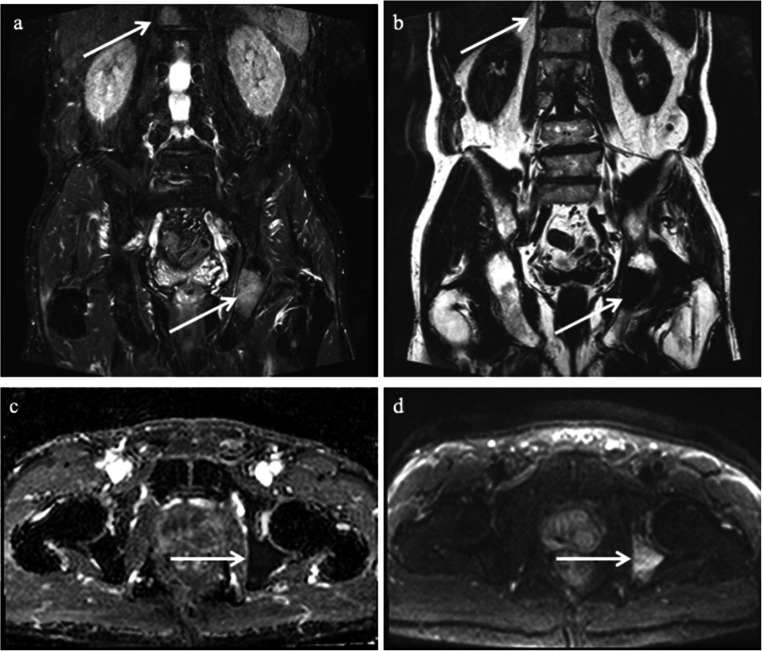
An 81-year-old patient with cT2/radT3a, PSA 9.5 ng/ml, and ISUP 3. The arrows indicate bone metastases in the left ischial body and the 12^th^ thoracic vertebral body. The prostate biopsies demonstrated a 90% Gleason grade 4 pattern in all ten biopsy cores. On reappraisal by a second uropathologist, this case was considered ISUP 4. a Coronal T2_tse_dixon water, (b) coronal T2_tse_dixon fat, (c) axial ADC map, (d) axial DWI b800 s/mm^2^
